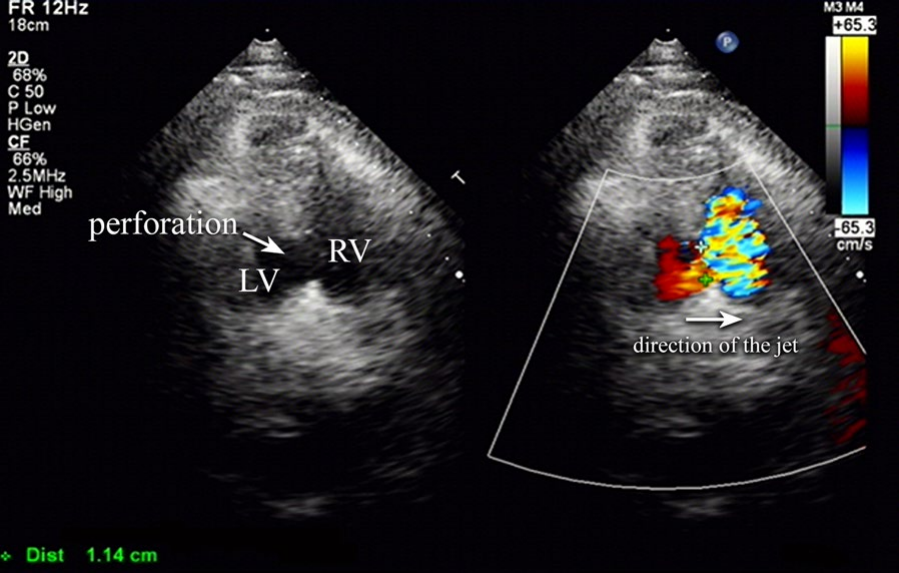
Transthoracic echocardiography: ventricular short-axis view with (right) and without (left) color-Doppler. A left-to-right shunt was detected in the level of ventricular level. The left ventricle is on the left-hand side (LV), so in the right ventricle (RV), a colorful signal of blood flow (downstream of the jet) can be displayed. The arrow on the left shows the location of the VSR, which is in dark color, and the arrow on the right denotes the direction of the jet. The Qp/Qs was 2.905 after the calculation
